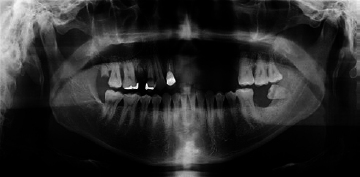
Postoperative panoramic radiograph (case 1).
